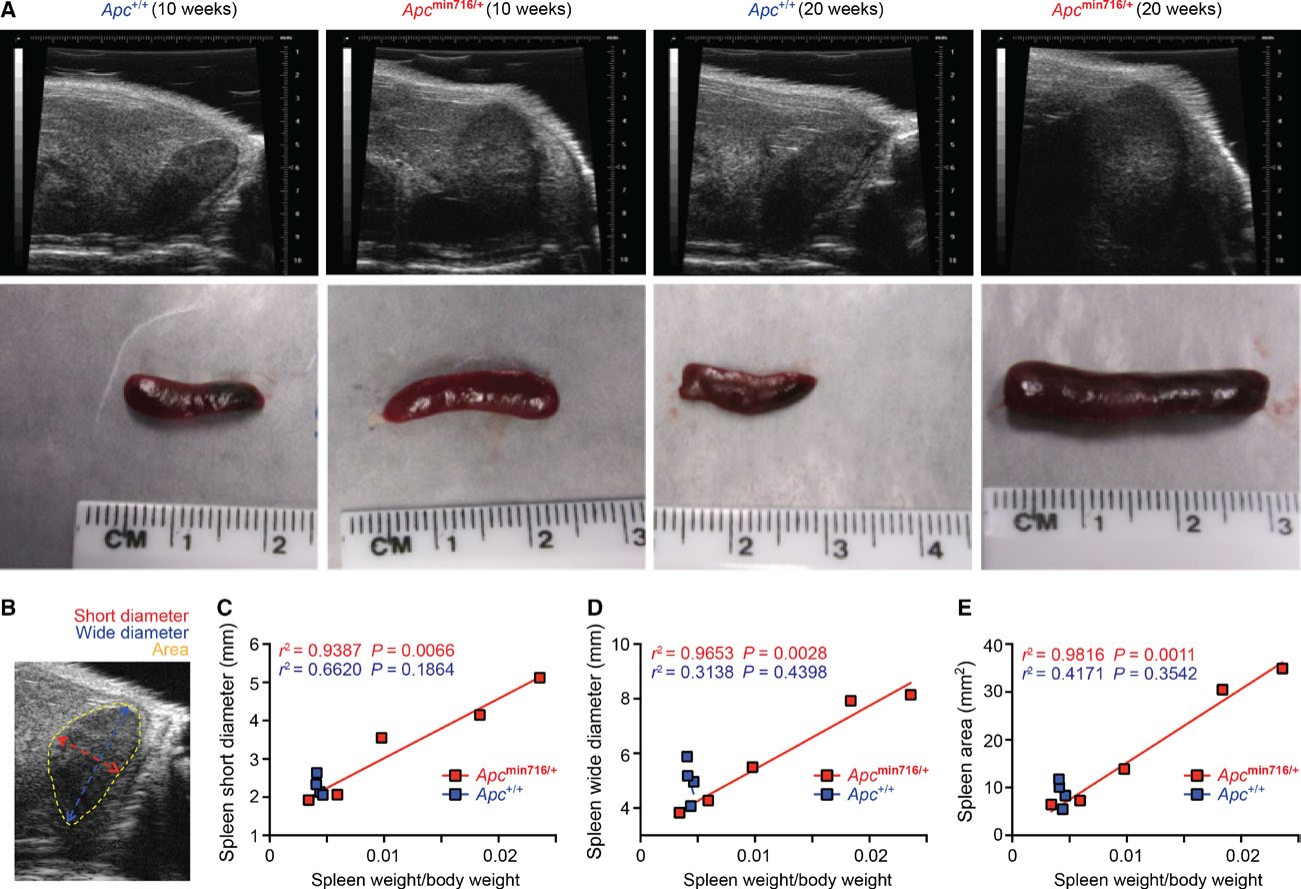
Dimensions of spleens from ultrasound images are correlated with spleen weight. (A) Ultrasound cross‐sections of spleens were imaged in vivo from 10‐week‐ and 20‐week‐old *Apc*
